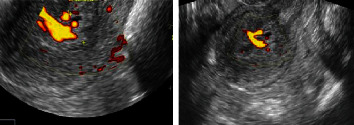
Transvaginal ultrasound screening.
